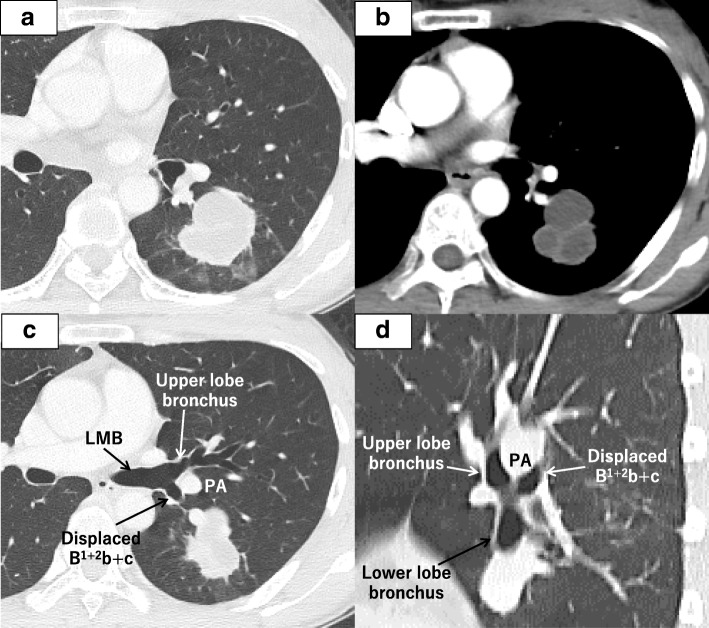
Chest computed tomography. There is a multicystic mass with a diameter of 35 mm on the dorsal interlobar parenchyma between the S^1+2^ and S^6^ segments in the left lung (a, b). The displaced bronchus arises from the left main bronchus and passes to the dorsal side of the left main pulmonary artery (c, d). LMB, left main bronchus; PA, pulmonary artery
